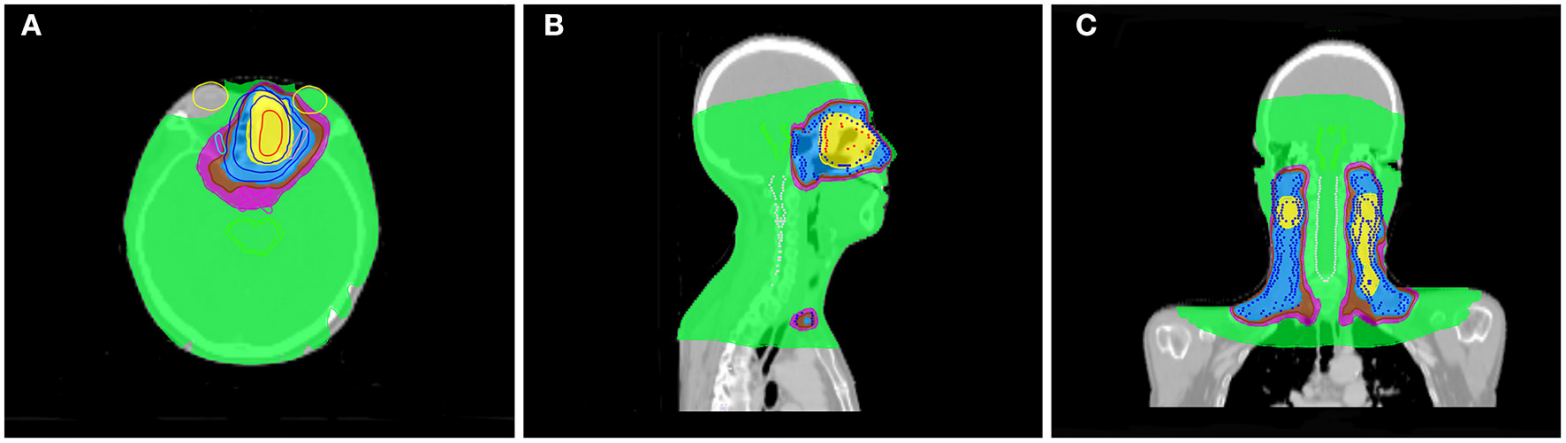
One patient distribution on Helical tomotherapy. Color-wash areas:60 Gy (yellow), 54 Gy (blue), 50 Gy (brown), 45 Gy (purple), 9 Gy (green). (A) refer to axial CT, (B) refer to sagittal CT, and (C) refer to coronal CT.
